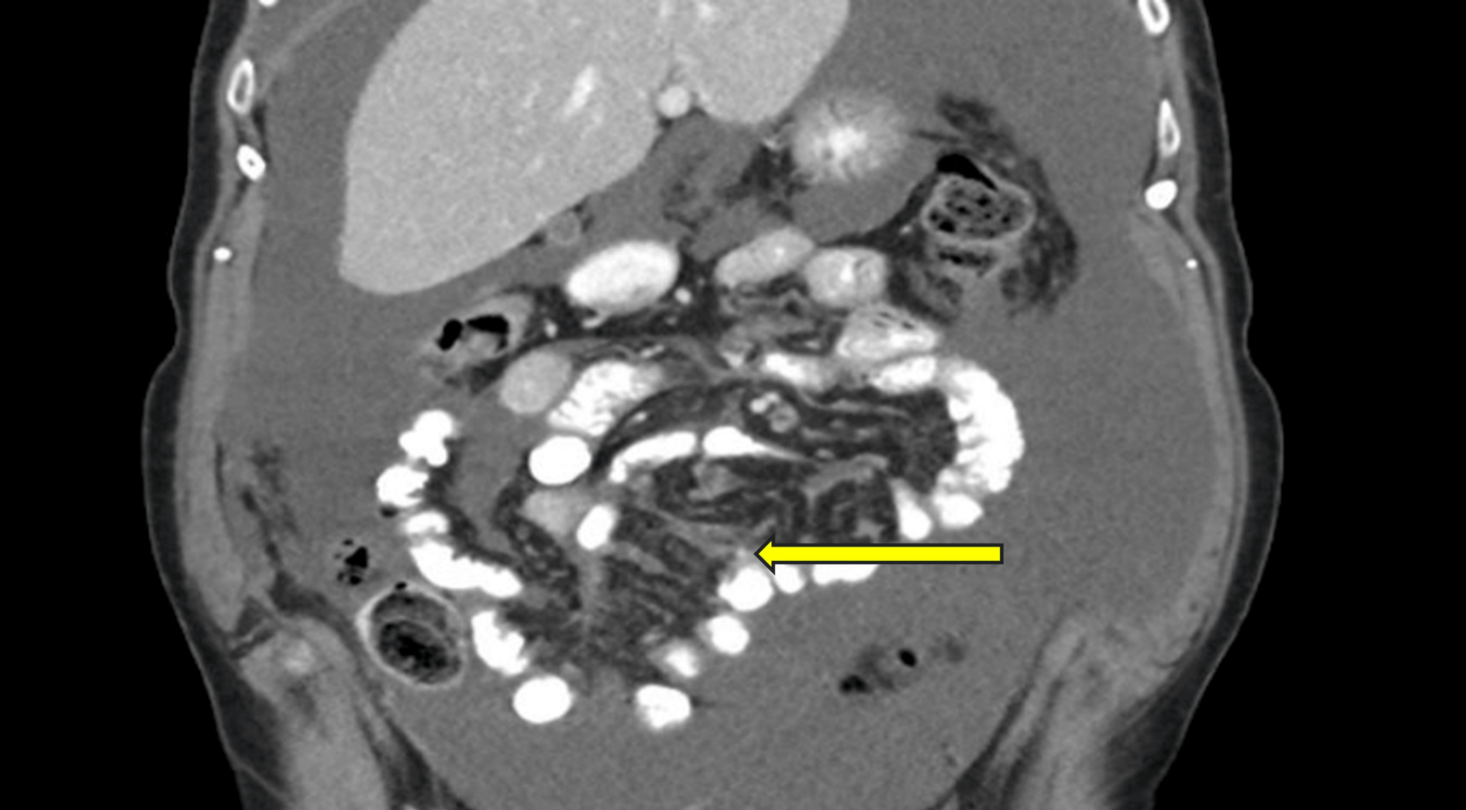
CT abdomen coronal section showing peritoneal nodules (yellow arrow)
